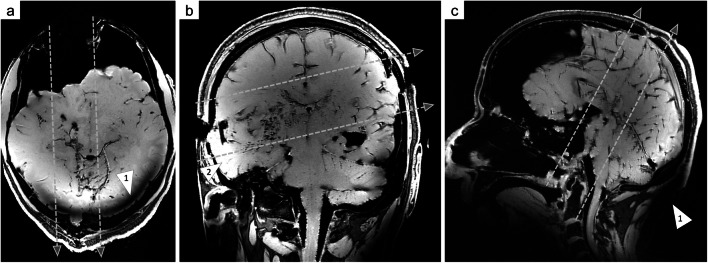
Multiplanar reformats of a spoiled 3D GRE sequence aligned to the individual bullet path (bullet trajectory: dashed arrows). This 7 T MRI sequence provided a good compromise between visualization of bone damage and soft tissue injuries. Case 1 (a: transversal view) demonstrated a transverse trajectory (entrance wound: frontal bone; exit wound: occipital bone), case 2 (b: coronal view) presented a horizontal trajectory (entrance wound: right temporal bone; exit wound: left parietal bone), and case 3 demonstrated a vertical trajectory (entrance wound: submental soft tissue; exit wound: parietal bone). B_1_ inhomogeneity was visible as brightened structures far from the image center (a: arrowhead 1). Signal dropouts (c: arrowhead 1) were observed in the lower occipital region because the field of view was smaller due to the absence of a body coil and B_1_ inhomogeneity
